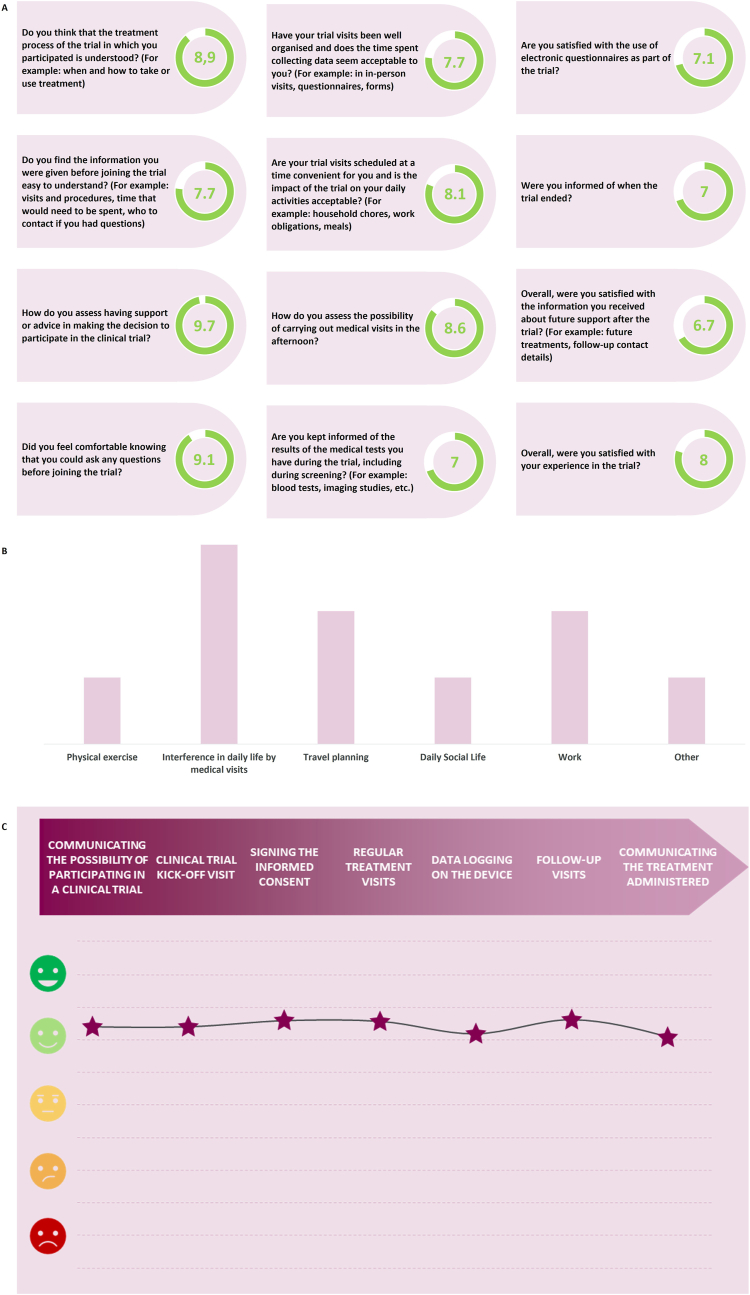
Quantitative results of the collection of the *Subject's Voice* data within CARABELA-CT. A. Subject satisfaction survey on clinical trial experience. B. Subject life areas affected as a result of participating in a CT. C. Empathy map showing the subject's emotional journey throughout the possible phases of a CT. This map shows the average of the feelings shared by the participants. CT: clinical trial.
